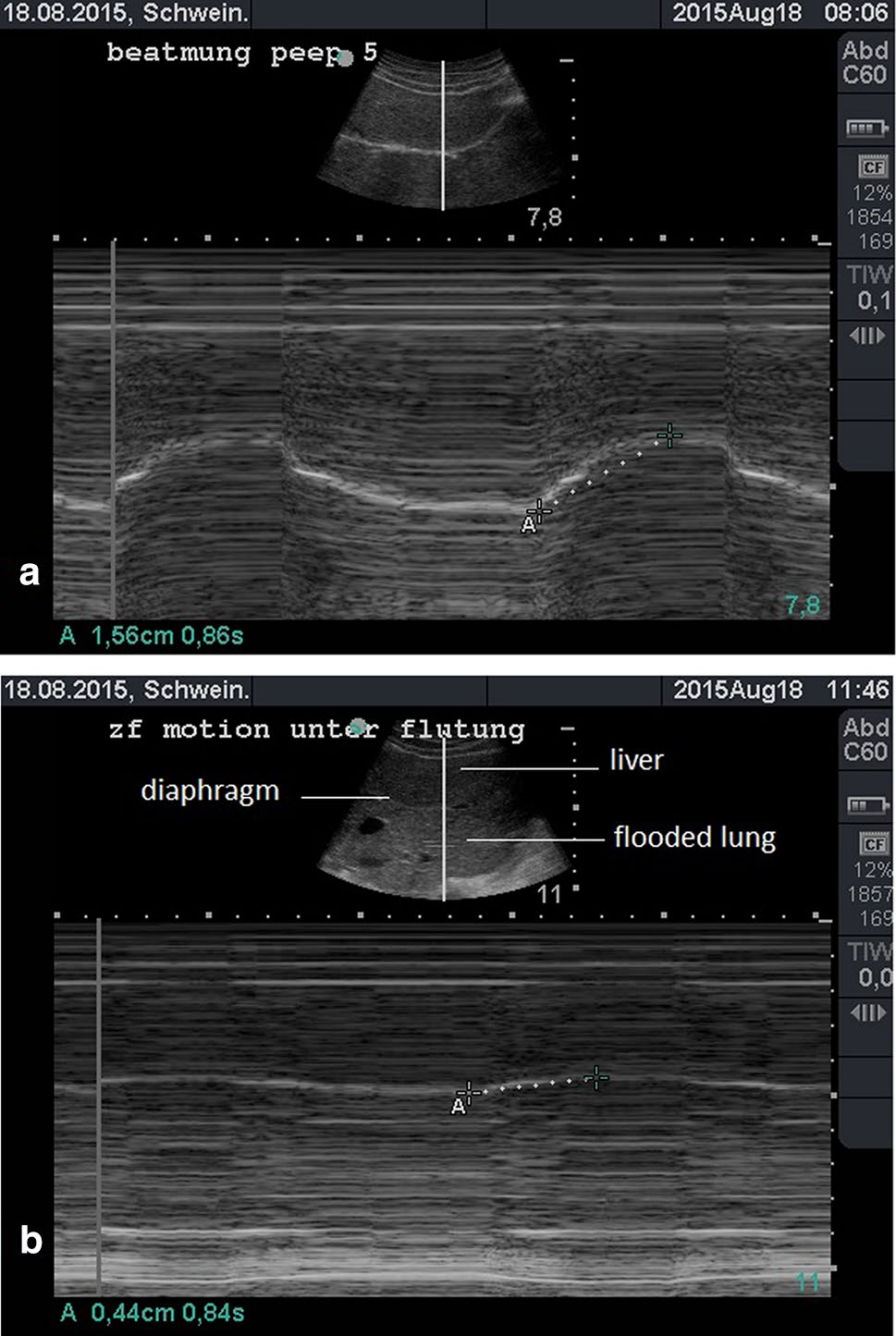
M-mode sonography for the measurement of diaphragm movement (end-expiration/end-inspiration difference) a during mechanical ventilation and b after lung flooding
